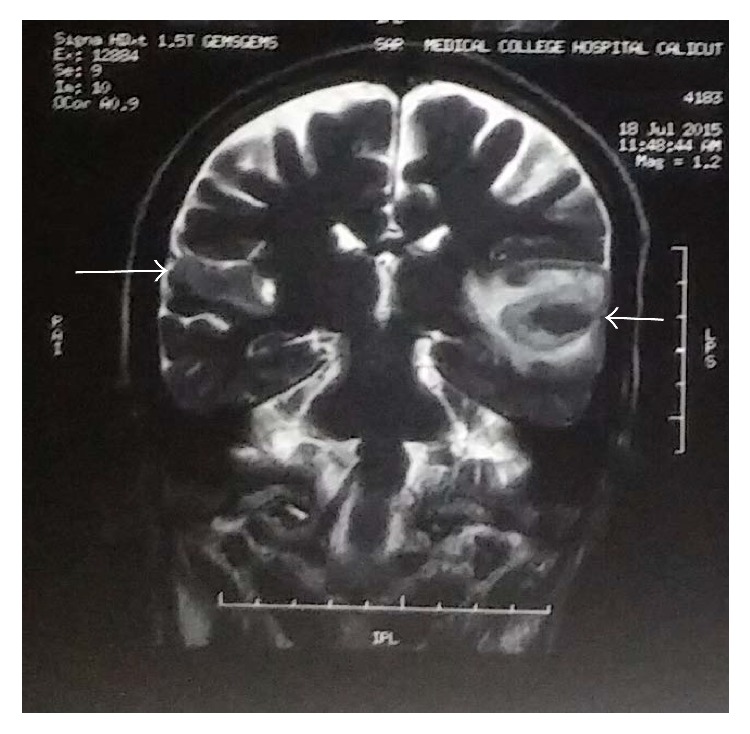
MRI brain showing infarcts in bilateral Heschl gyri.
